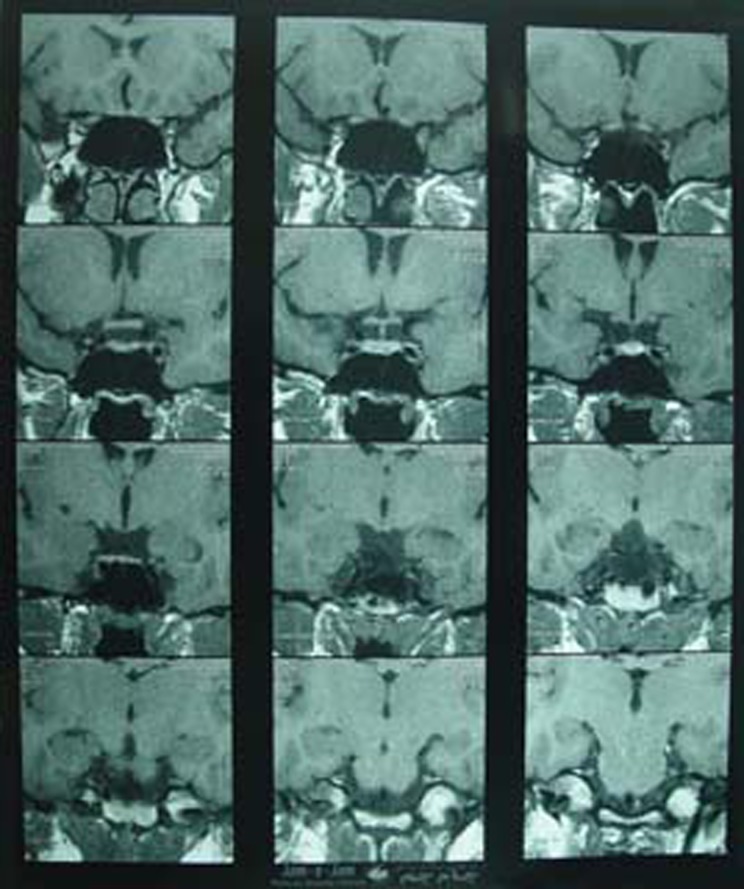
The MRI of patient’s brain and pituitary gland that shows no pituitary tumor
